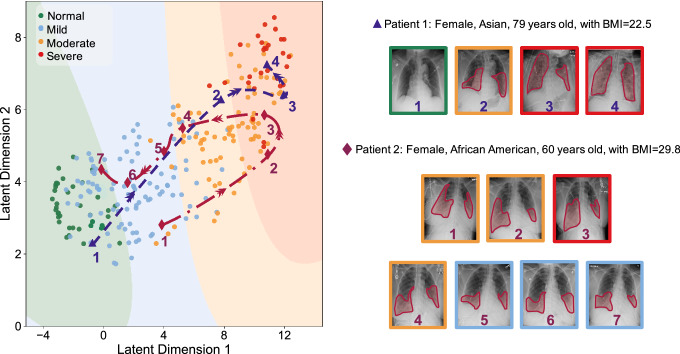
Latent space representation of the X-rays. The dimensionality of the penultimate layer of the network is reduced using UMAP, and the resulting latent space is clustered into 4 regions corresponding to different severity degrees using GMM. Green, blue, orange, and red are related to the normal, mild, moderate, and severe classes, respectively. The datapoints for each class are shown as circles with the corresponding color. The progressions of the disease over time for two patients are shown by the dashed blue (following a progression path) and red (following a recovery path after progression) paths in the latent space. Image segmentation is used as an indication of areas of interest and they are not necessarily quantitative measures for the severity (see Methods for more details about the severity assessment).
